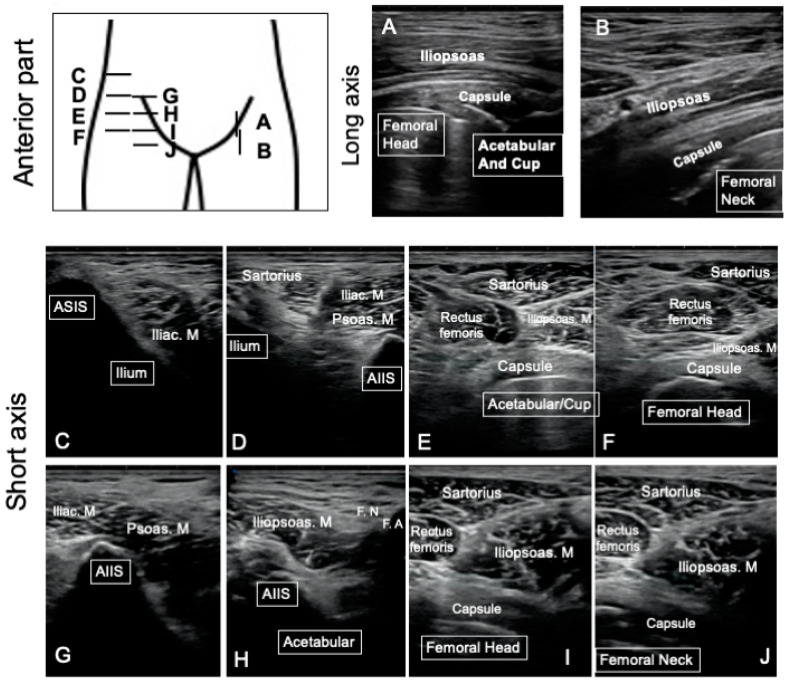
Representative sonography images of the anterior part in hip resurfacing patients. Periprosthetic hip soft tissues of the anterior part were assessed by longitudinal (A,B) and short axial (C–J) images. In the longitudinal images, the iliopsoas and capsule in front of the acetabular, cup, resurfacing head, and femoral neck were observed (A,B). In the short axial images (C–J), the iliac and psoas muscle, sartorius, rectus femoris, and capsule were observed using the anterior superior iliac supine (ASIS) (C), anterior inferior iliac supine (AIIS) (D,G,H), femoral nerve (F.N.), and femoral artery (F.A.) (H) as bony landmarks.
